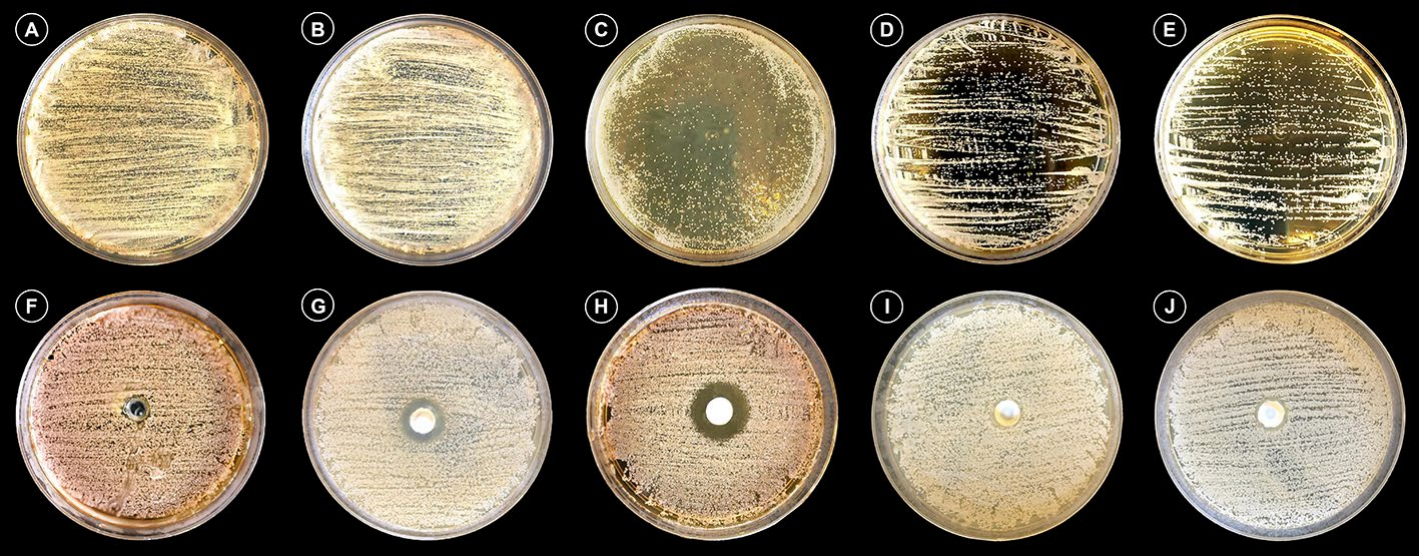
Antifungal analysis considering the adherence method (a–e) and the agar-well diffusion method, as well as the zone of inhibition (f–h): (a, f) negative control (distilled water); (b, g) CT; (c, h) CTCh; (d, i) EX; (e, h) EXCh.
